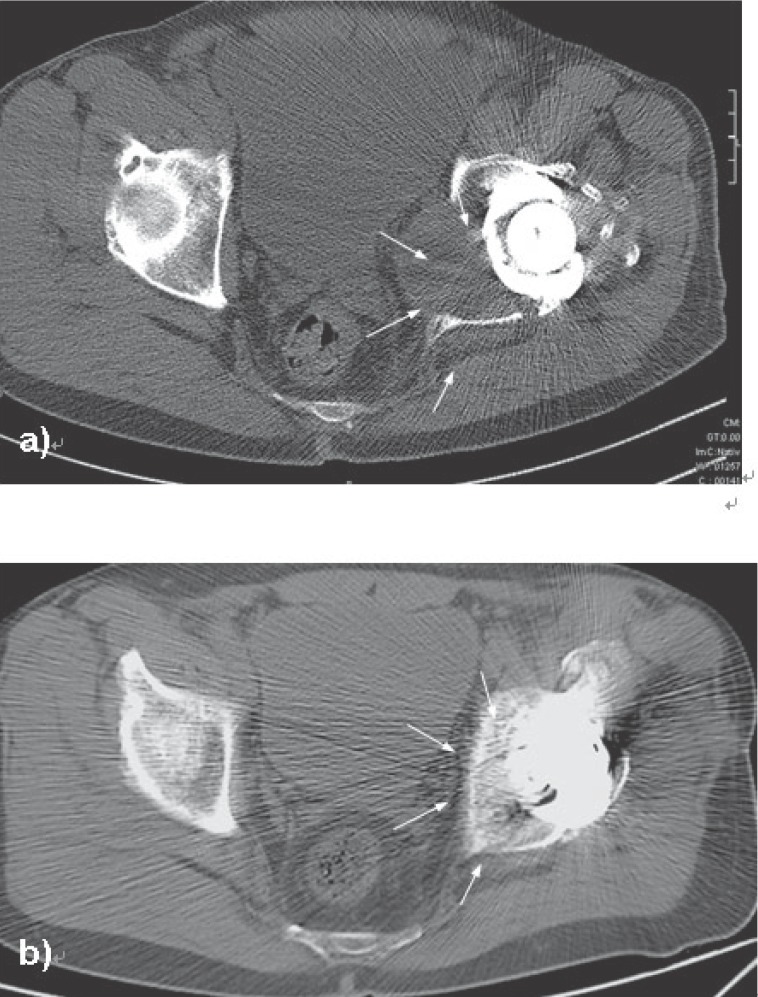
CT-scan before (a) and six months after (b) implantation of a BMP2/MSC-composit which was augmented by autologous and allogen spongious bone into an osteolytic pelvic cavity. a) The wear debris induced pelvic osteolysis lead to a pseudo-tumour with inflammative soft tissue. The inner cortical wall behind the cup is completely destructed whereas a cortical ring allows still a sufficient fixation of the thread cup. As a result to foreign body reaction ipsilateral pelvic organs are shifted by inflammatory tissue. The border of the expanding pseudotumour is marked by arrows. b) Six months after cuerettage of the pseudo-tumour and implantation of BMP-2/MSC-composit the initial critical size osteolysis is replaced by bone.
